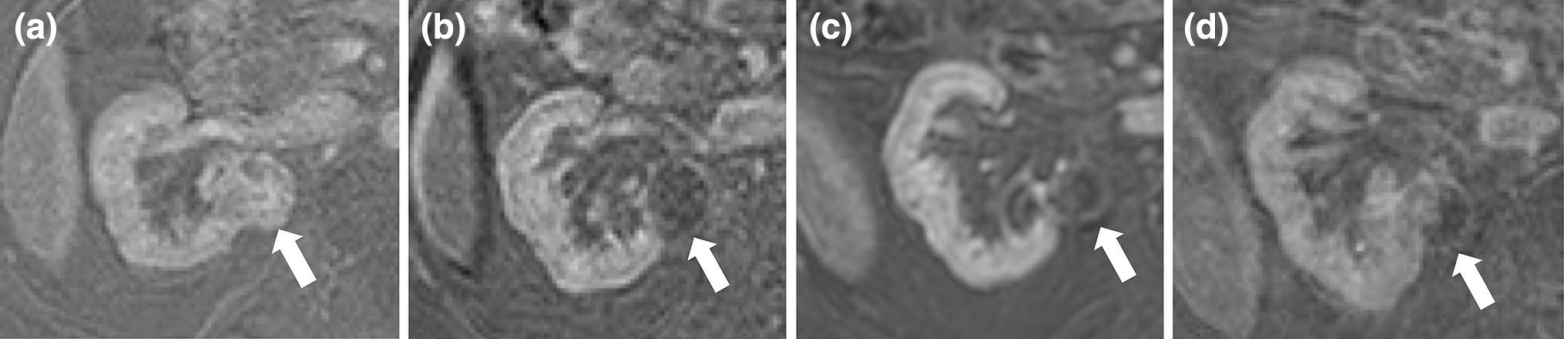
Tumor recurrence after cryoablation of a RCC. a Pre-ablation fat-suppressed T1-weighted CE MRI shows a 3.0 cm enhancing mass in the mid portion of the right kidney, posterior to the renal vein (arrow). b, c Follow-up CE MRI 6 months and 1 year after cryoablation show lack of enhancement within the ablation zone (arrows). d Follow-up CE MRI 2 years after cryoablation. In the ablated area (arrow) there is lack of enhancement medially and new enhancement in the lateral aspect consistent with tumor recurrence
